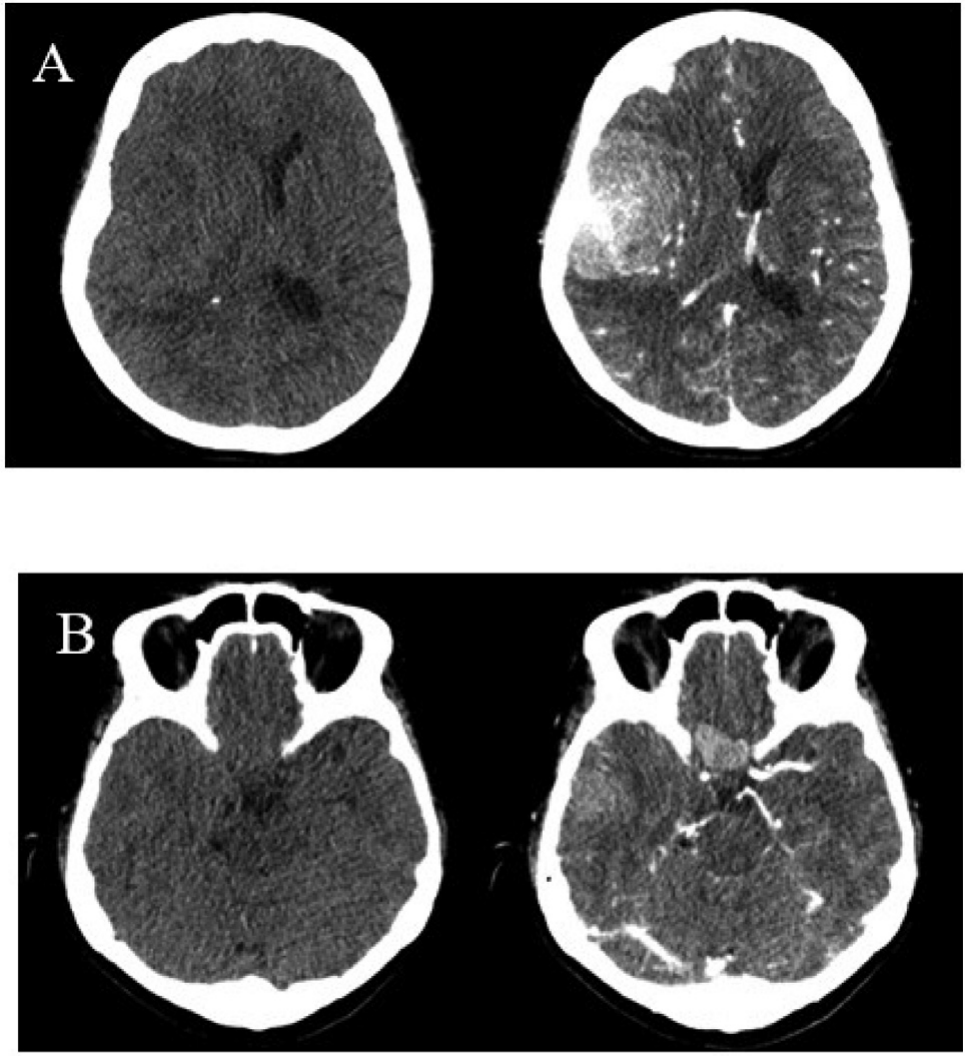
Head CT scan axial section showed inhomogeneous isodense mass in the frontotemporoparietal region dextra and extending into the surrounding (A), an inhomogeneous isodense mass in the right temporalis and suprasellar region (B), enhanced after contrast administration (A, B).
